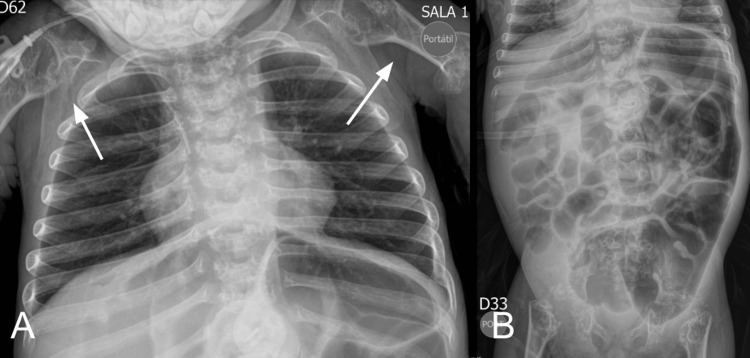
Chest X-ray (anteroposterior view) and abdominal X-ray (anteroposterior view) Chest X-ray (anteroposterior view) (A) shows characteristic punctuated sclerotic bone lesions in the epiphysis with multiple punctate, sclerotic cartilaginous calcifications distributed in all visualized osseous structures, particularly prominent at the epiphyses. There is rhizomelic shortening of the humeri, with evident reduction of the proximal segment length, associated with mild metaphyseal flaring of the distal humerus (white arrows). Cortical outlines are preserved, and no pathologic fractures are identified. The ribs demonstrate normal morphology, and no pulmonary parenchymal abnormalities, pleural effusion, or mediastinal enlargement are observed. Abdominal X-ray (anteroposterior view) (B) shows moderate gaseous dilatation of multiple bowel loops, without evidence of pneumatosis intestinalis, portal venous gas, or free intraperitoneal air. The osseous structures demonstrate diffuse punctate cartilaginous calcifications involving the pelvis, femora, vertebrae, and iliac bones. Both femora show significant rhizomelic shortening with mild metaphyseal flaring. The acetabular roofs are poorly developed bilaterally, suggestive of associated developmental dysplasia of the hips. Sacroiliac joints and pubic symphysis are preserved. The vertebral column demonstrates adequate alignment without congenital segmentation anomalies.
